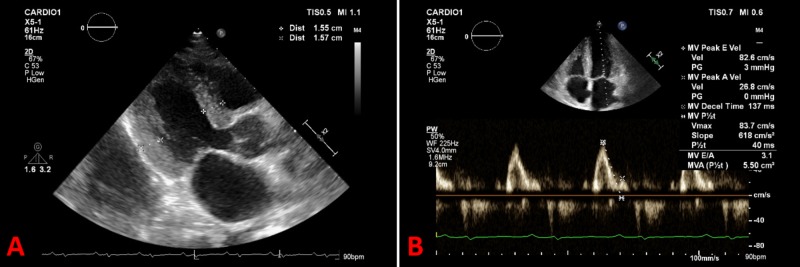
Cardiac ultrasound Cardiac ultrasound showing: A) left ventricular hypertrophy; B) restrictive left ventricular filling pattern with E/A ratio 3.1 and E/e' ratio 20.
